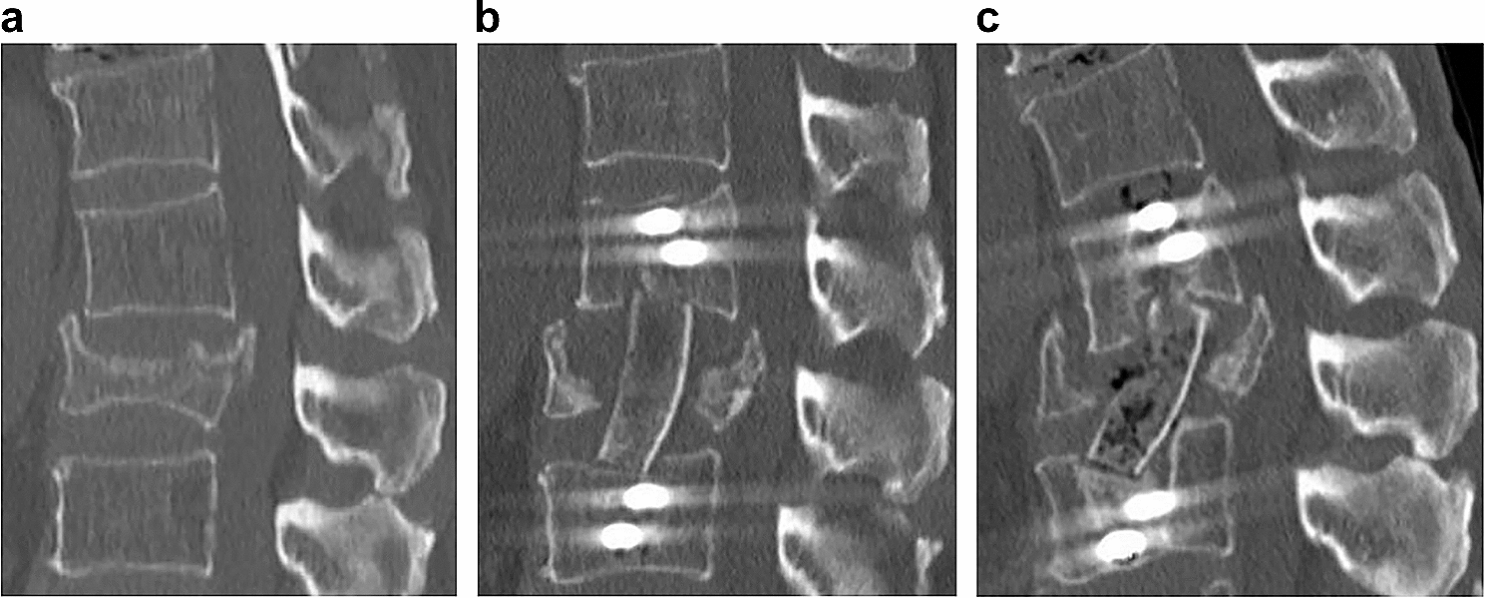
CT image reconstruction of the anterior column in a spinal fracture patient with maxVB = 0. a A 76-year-old male sustained an A3-type vertebral fracture at L2 after coughing. b The anterior column was reconstructed with the iliac bone and the L1-L3 vertebral body was fixed with unilateral side screws and a rod. c No bony fusion was achieved postoperatively
